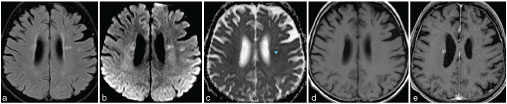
Brain MRI. (a) Axial slice of *T*
